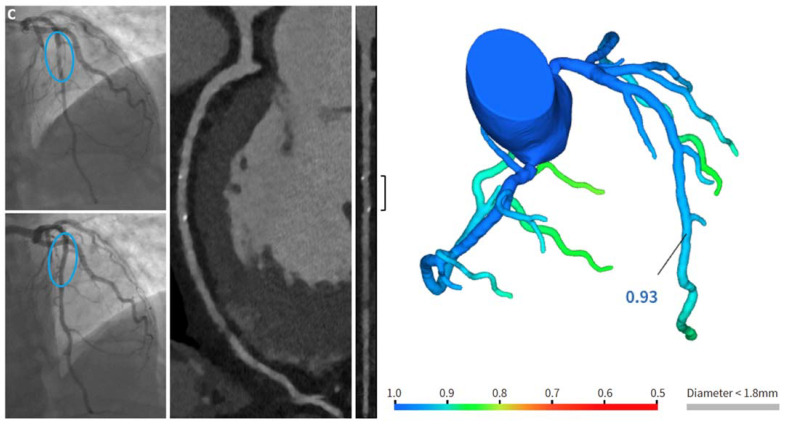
Examples of completely reabsorbed RMS.Each case (A–C) shows two angiographic images (on the left, from top to bottom: before and after RMS implantation), two CCTA reconstructions (from left to right: curved multiplanar reconstruction and straightened vessel view), and a CT-FFR image. The blue circle in the angiographic images indicates the target segment before and after treatment with RMS. The bracket in the straightened CCTA reconstruction indicates the coronary segment covered with RMS.
